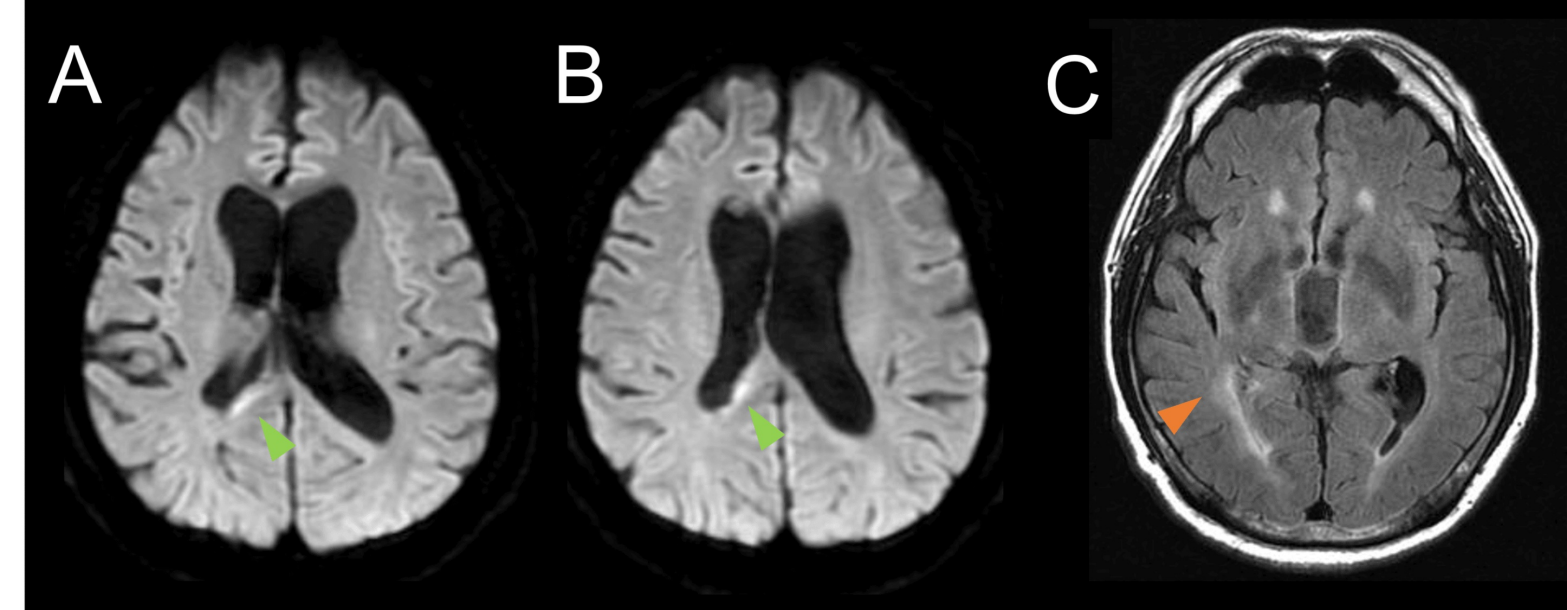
Brain MRI on day 93. Follow-up diffusion-weighted imaging on day 93 confirmed the resolution of intraventricular pus. However, enlargement of the lateral ventricles and a slightly high signal around the right lateral ventricle persisted (green arrowheads). The fluid-attenuated inversion recovery sequence revealed a narrowing of the inferior horn of the right lateral ventricle, suggestive of adhesions (orange arrowhead).
